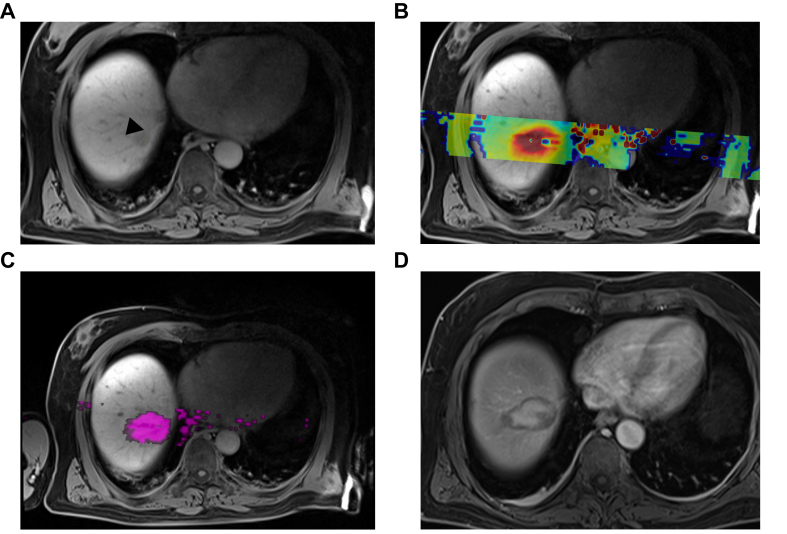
Case example. (A) Axial T1w images after injection of gadoxetic acid shows a hepatocellular carcinoma lesion (arrowhead) in Segment 8. (B) Thermometry image shows MR-thermometry predicted temperature within the liver during the ablation. (C) Thermal dose image shows the volume exposed to lethal dose of thermal energy with a volume of 22,734 mm^3^. (D) Portal phase MRI image on the postprocedural first day shows the ablation zone with a volume of 21,349 mm^3^, which shows excellent similarity with thermal dose in terms of shape.
